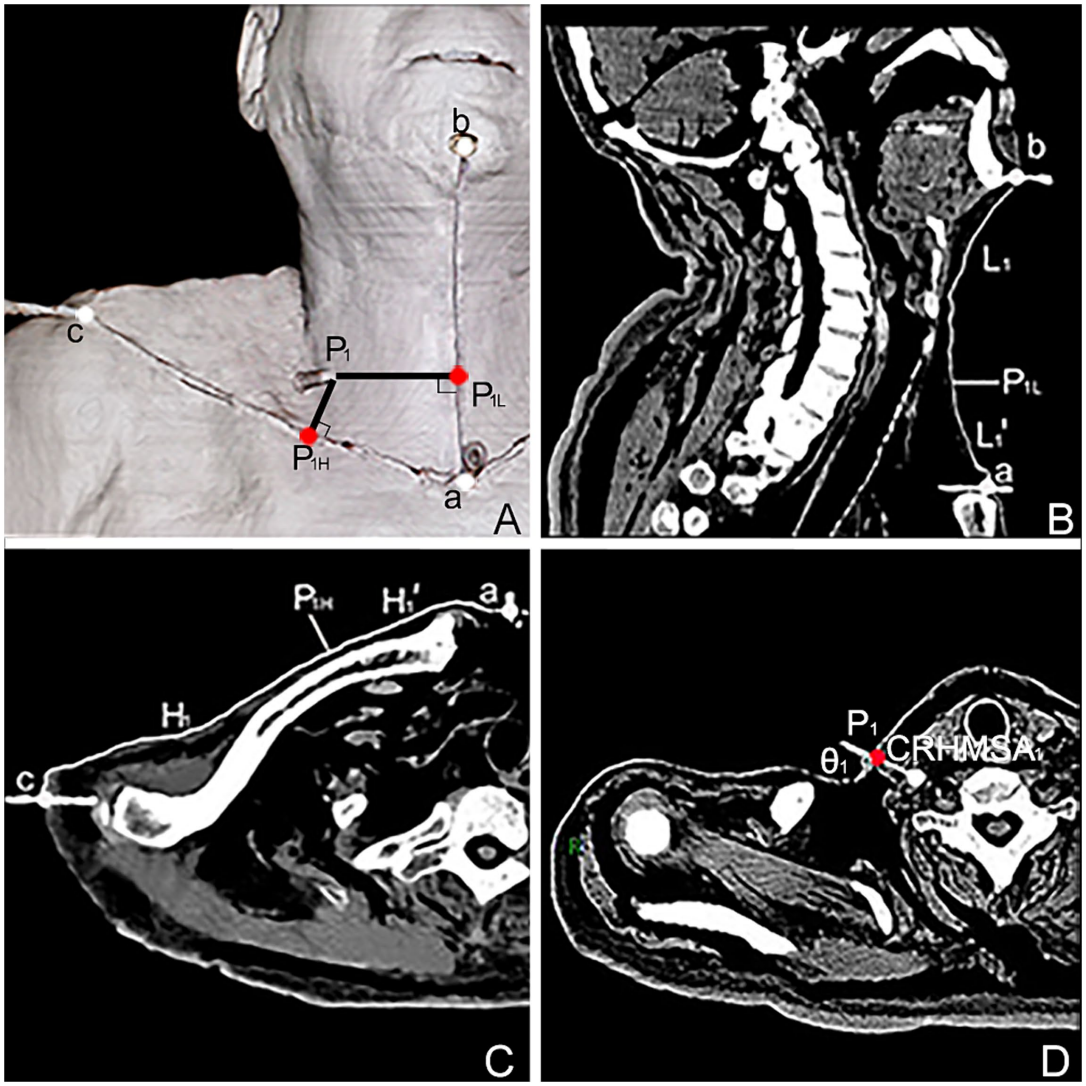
Spiral CT localization image of the center of the highest region of muscle spindle abundance (CRHMSA1) of the right SA muscle in males. (A) Three-dimensional reconstructed spiral CT image showing the position of CRHMSA1 on the body surface and the designed reference line. P1 is the surface projection point of the SA muscle CRHMSA1. P1H = intersection point generated by creating a line perpendicular to line H through P1. P1L = intersection point of the H-line passing through P1 and the L-line. a-P1H=H1’, a-P1L = L1’. (B) Lengths of the L and L1 lines in the coronal plane were measured. (C) Measure the lengths of the H and H1’ lines on the section passing through line H. (D) Determine the depth and angle of needle insertion for CRHMSA1 in the cross section.
